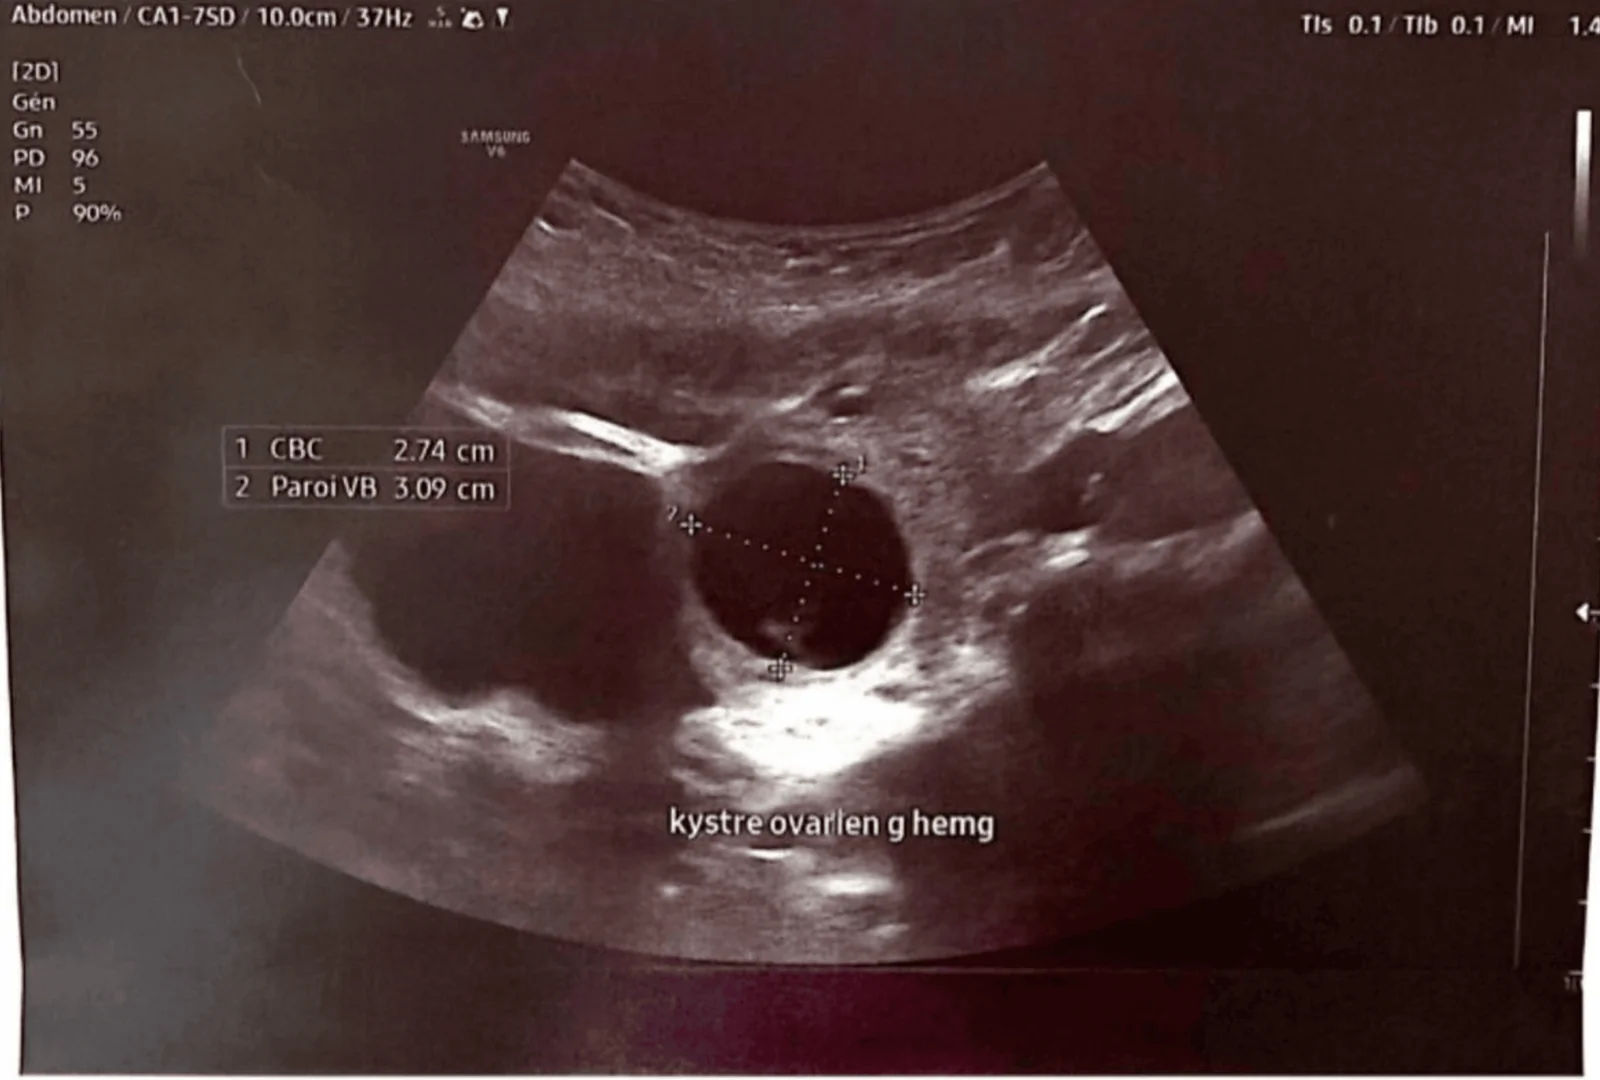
Suprapubic ultrasound showing a left ovarian cyst Suprapubic ultrasound image showing a left ovarian cyst measuring approximately 2.74 × 3.09 cm. The cyst appears unilocular and anechoic, with a thin wall and posterior acoustic enhancement, consistent with a functional ovarian cyst. Translation of text at the bottom of the figure: “Left hemorrhagic ovarian cyst." Other text visible on the figure: "CBC" refers to the largest cyst measurement; “Paroi VB" refers to the bladder wall.
